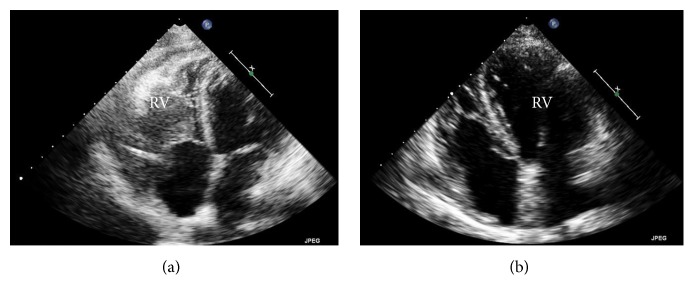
Pre- and posttransthoracic echocardiogram images. (a) Apical four-chamber view demonstrating a large mass in the right ventricle with extension into the right atrium. (b) Apical four-chamber view demonstrating complete resolution of the mass after chemotherapy.
